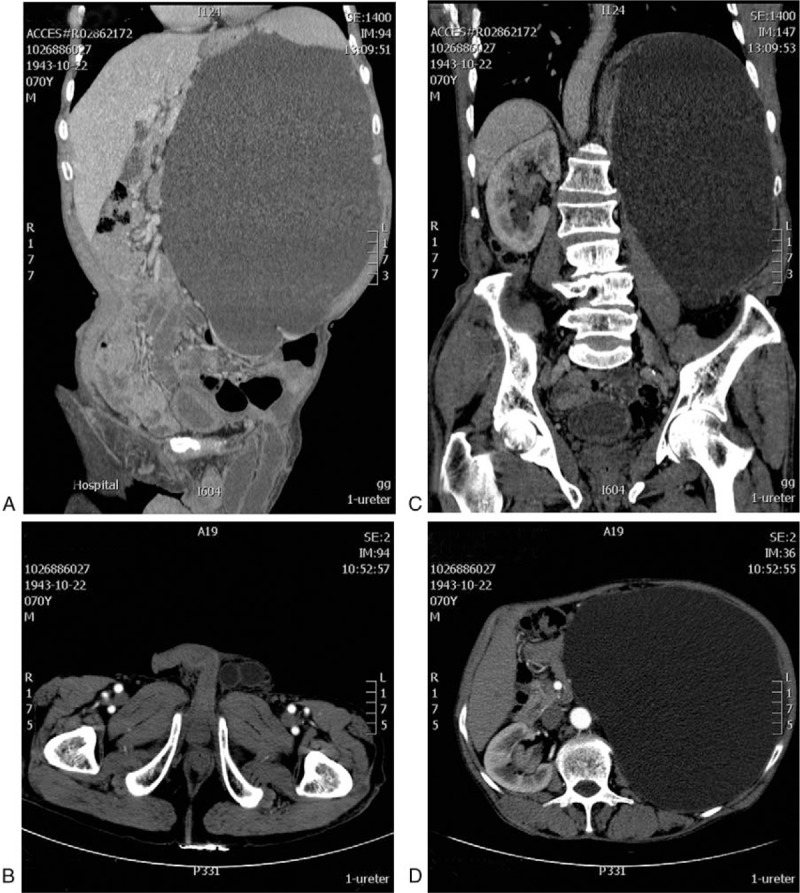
CT images of a 70-year-old patient with a left uretero-inguinal hernia, left hydronephrosis, and right renal pelvic carcinoma. (A) The coronary CT shows a ureteral loop in the inguinal canal and severe hydronephrosis of the left kidney. (B) The dilated ureter in the inguinal canal is shown on the axial CT. The coronary (C) and axial CT (D) show the dilated pyelocaliceal system and a mass in the right renal pelvis. CT = computed tomography.
